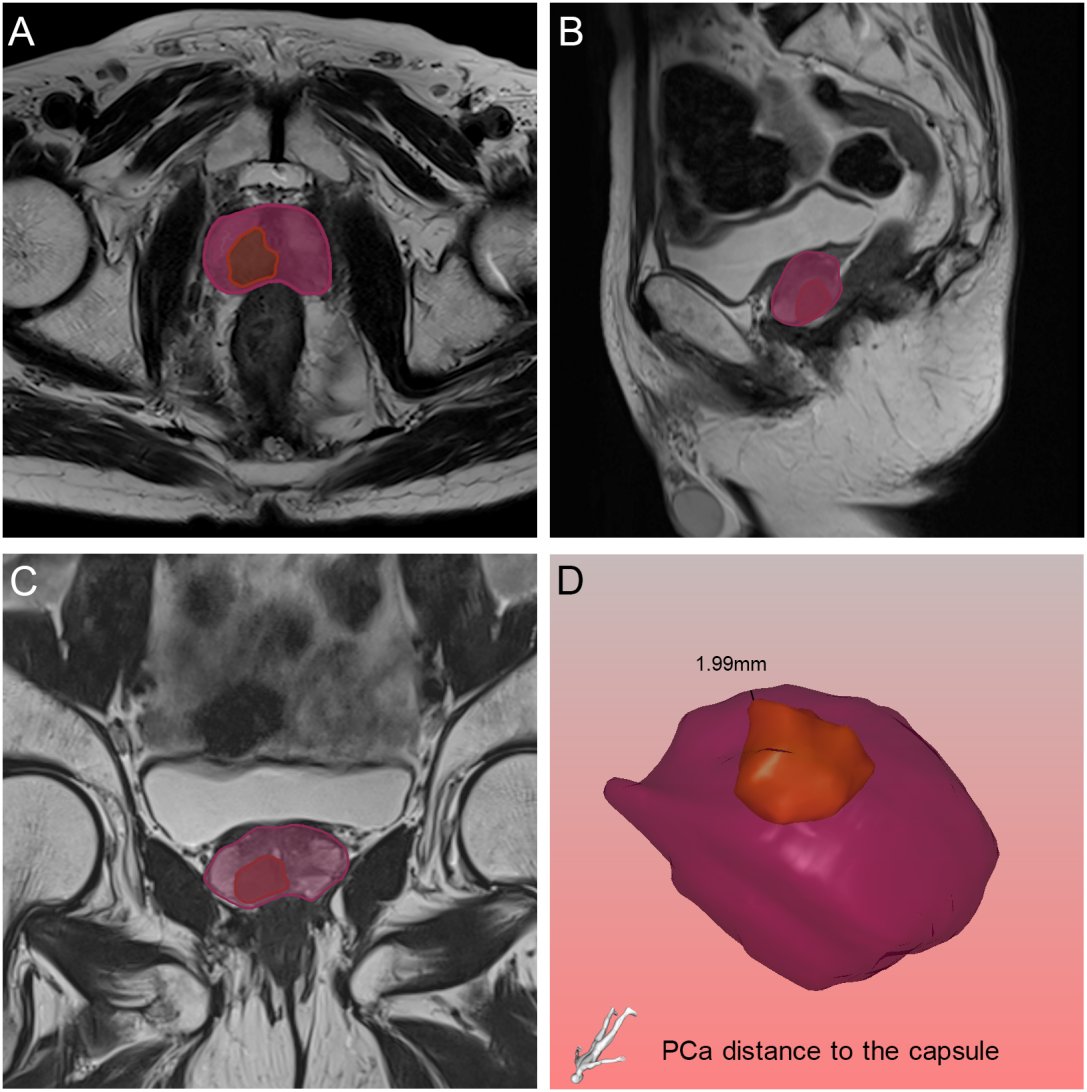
Relative position of the tumor to the capsule in axial (A), sagittal (B) and coronal (C) views on magnetic resonance imaging, and the black line demonstrated the PCa distance to the capsule found by the three-dimensional visualization technique (D). The orange and purple sections indicated the prostate cancer and prostate respectively.
